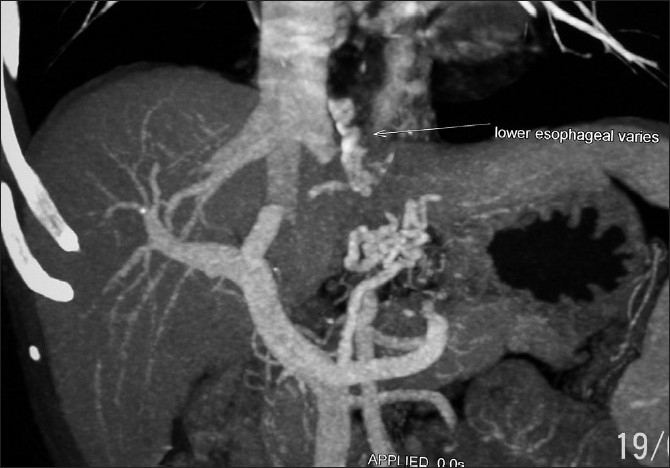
CT angiogram showing collaterals marked with arrows.
